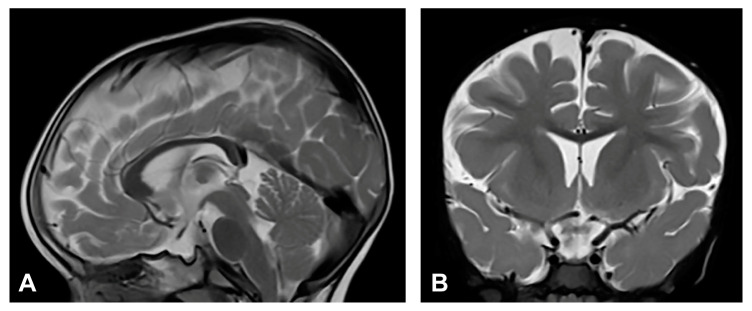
(Case 6) (A) Sagittal and (B) coronal T2W magnetic resonance images at the age of one and a half without pathological findings.
